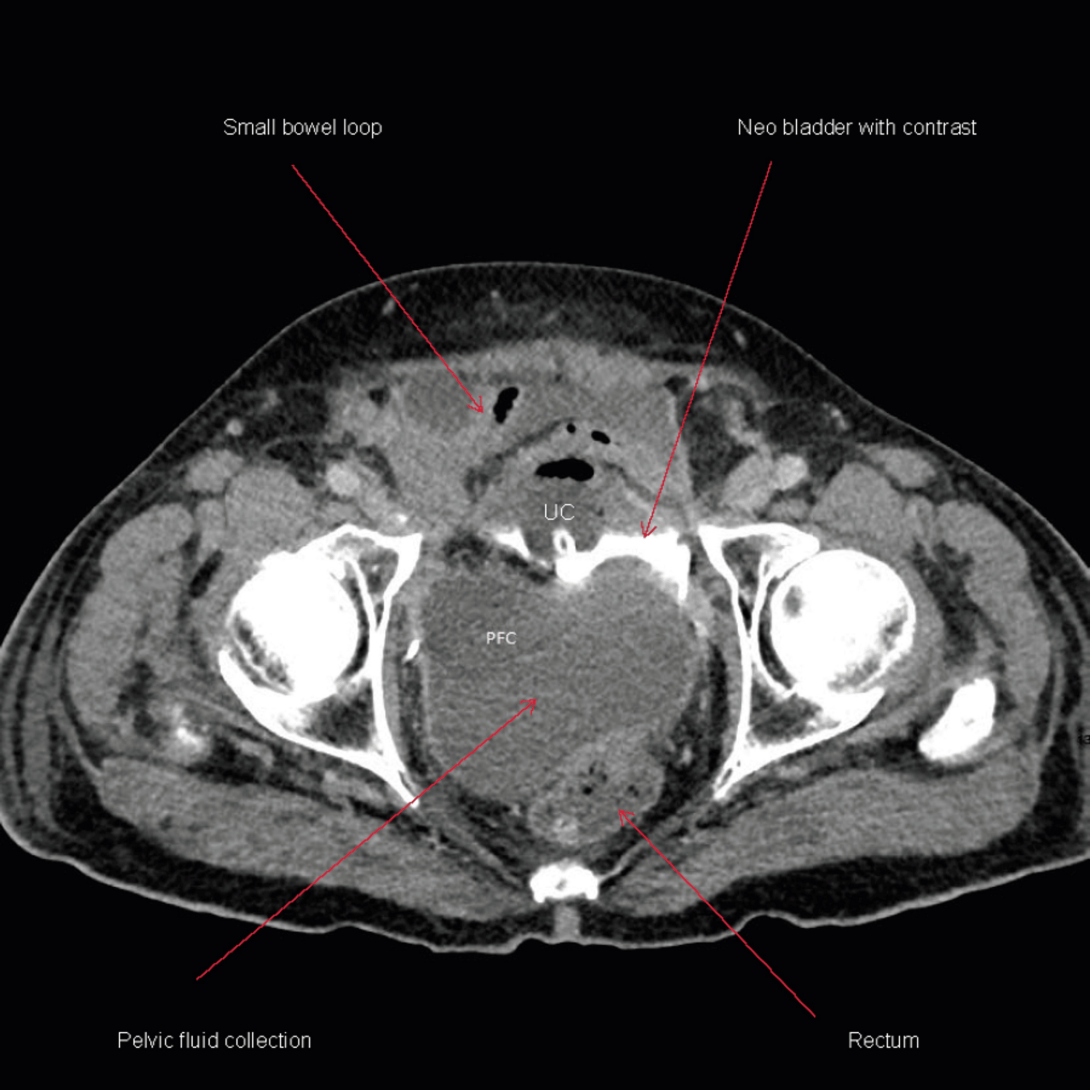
CT Abdomen and pelvis (axial view) with intravenous and oral contrast and cystographic phase three days after admission showing mild small bowel dilatation/distension and pelvic fluid collection PFC: Pelvic fluid collection
